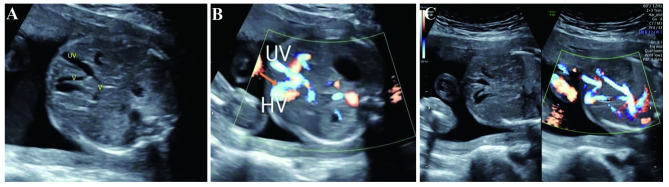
Ultrasonic images of type IIIA UPSVS (intrahepatic portal-systemic shunt, IHPSS) (A-C) A communicating branch connected the left portal vein with the middle hepatic vein. The arrow showed the communicating branch. *UV*: umbilical vein, *HV*: hepatic vein
